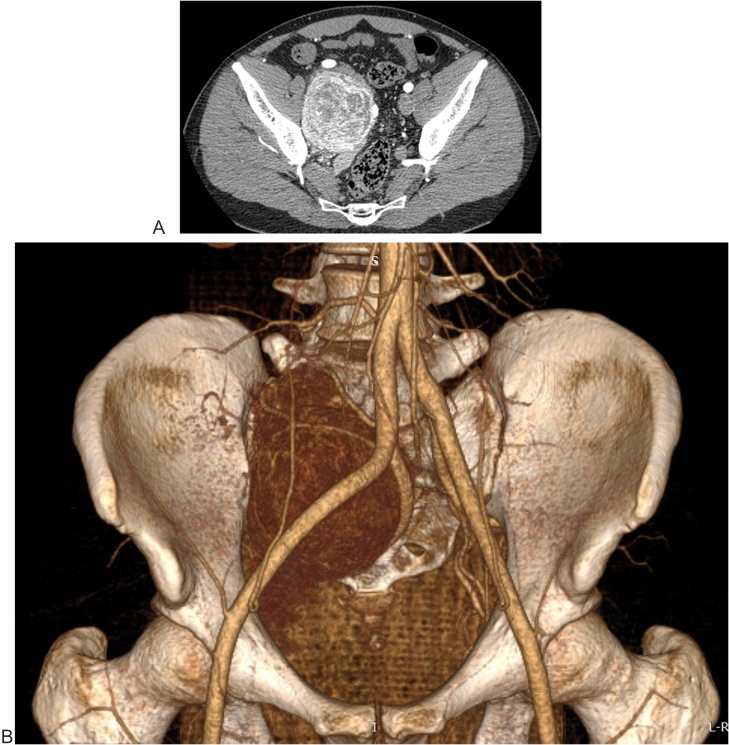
Contrast enhanced computed tomography of abdomen showing a large retroperitoneal mass.
